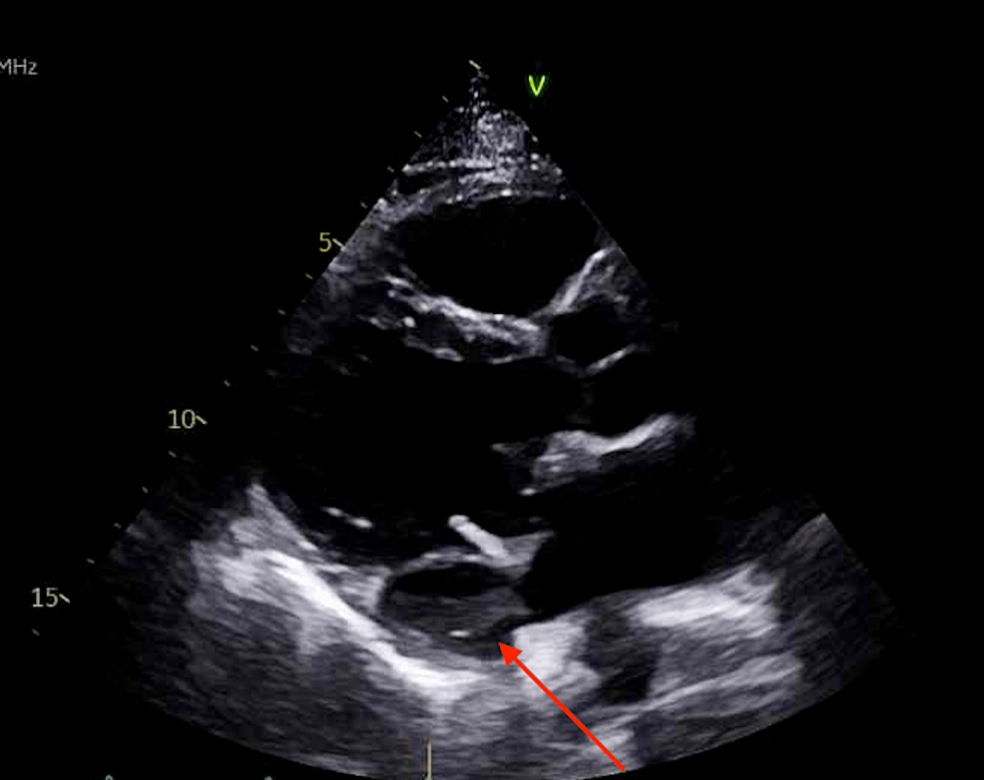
Transthoracic echocardiogram three months after mitral valve replacement. The red arrow indicates left ventricular pseudoaneurysm formation noted on transthoracic echocardiogram three months after mitral valve replacement.
